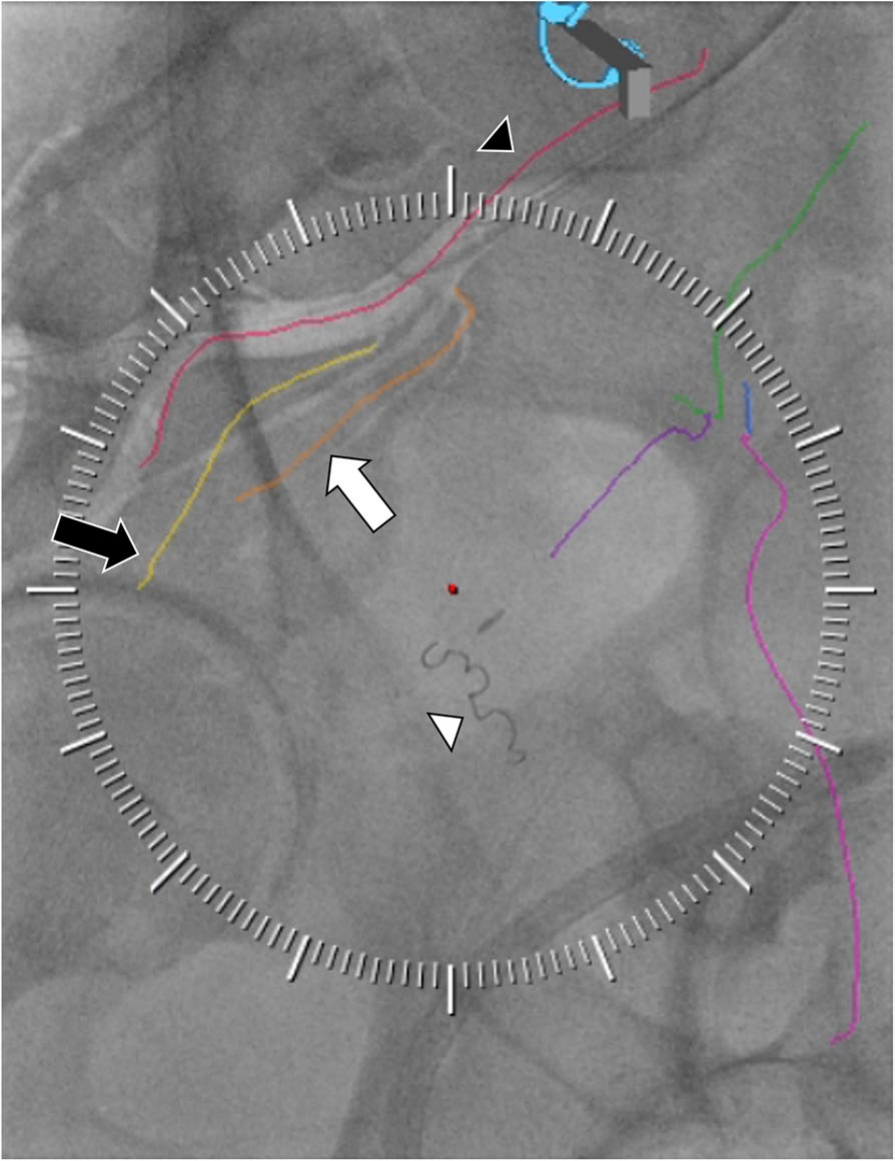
Fluoroscopic fade image of a right internal iliac artery angiogram in a steep ipsilateral oblique from patient 4 following left sided embolization, which included the use of a 2 mm x 4 cm coil (white arrowhead). Embolization guidance overlay lines traced from the PCD CTA and registered with 2D/3D fusion are shown: Red line (black arrowhead) traces the right internal iliac artery through the anterior division; Orange line (white arrow) traces the origin of the right prostatic artery from the vesicoprostatic trunk; Yellow line (black arrow) traces a right accessory prostatic vessel. The white lines arranged in a circle allow for adjustment of the overlays with the DSA image. An incidental dropped clip from prior cholecystectomy projects near the embolization coil
